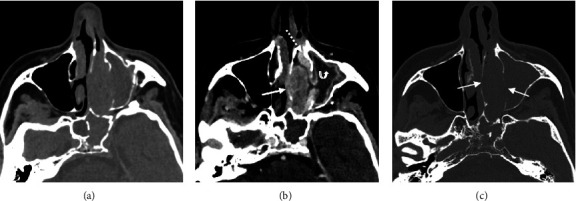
Axial view CT facial bone. (a) Noncontrast image demonstrating soft tissue density opacification of the left nasal cavity and left maxillary sinus with normal aeration of the right nasal cavity and maxillary sinus. (b) Postcontrast image revealing a heterogeneously enhancing mass within the middle left nasal cavity (solid arrow) with homogeneous enhancement of the inferior nasal turbinate (dashed arrow) which is displaced inferiorly and laterally. Mucosal enhancement of the left maxillary sinus with no enhancement of the contents of the left maxillary sinus (curved arrow) indicating retained secretions. (c) Bone reconstruction images showing bowing of the bony nasal septum and medial wall of the left maxillary sinus (solid arrows) which are reduced in density indicating chronicity with no features of bony destruction.
